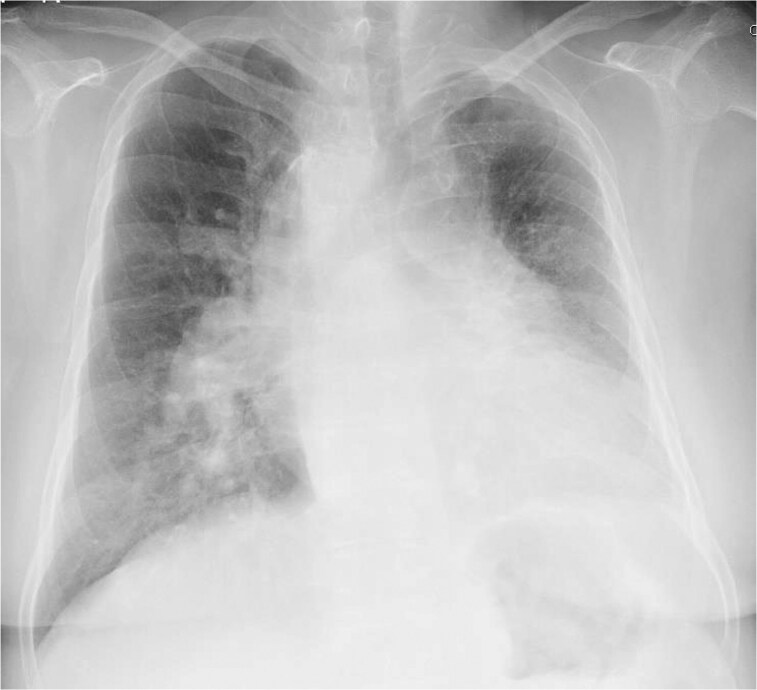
Chest radiography showing the right aortic arch and trachea deviated to the left, decreased left lung volume and right lung hyperinflation, dilated right pulmonary artery, and protrusion of the left second and fourth arches.
